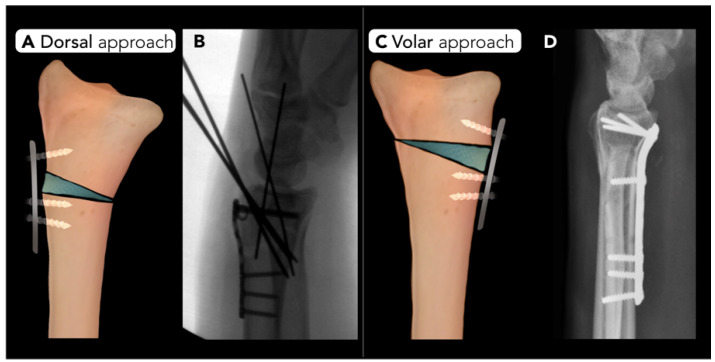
Distal radial corrective osteotomy. Two surgical approaches for correcting distal radius deformities. (A) Dorsal approach: An opening-wedge corrective osteotomy with plate and screw fixation to correct dorsal tilt. (B) Intraoperative lateral radiograph of the wrist shows the dorsal corrective osteotomy stabilized with a plate, screw, and K-wire. (C) Volar approach: An opening-wedge corrective osteotomy with plate and screw fixation is utilized to correct volar tilt. (D) Post-operative radiograph highlights the distal radial corrective osteotomy with plate and screw fixation addressing post-traumatic deformity.
